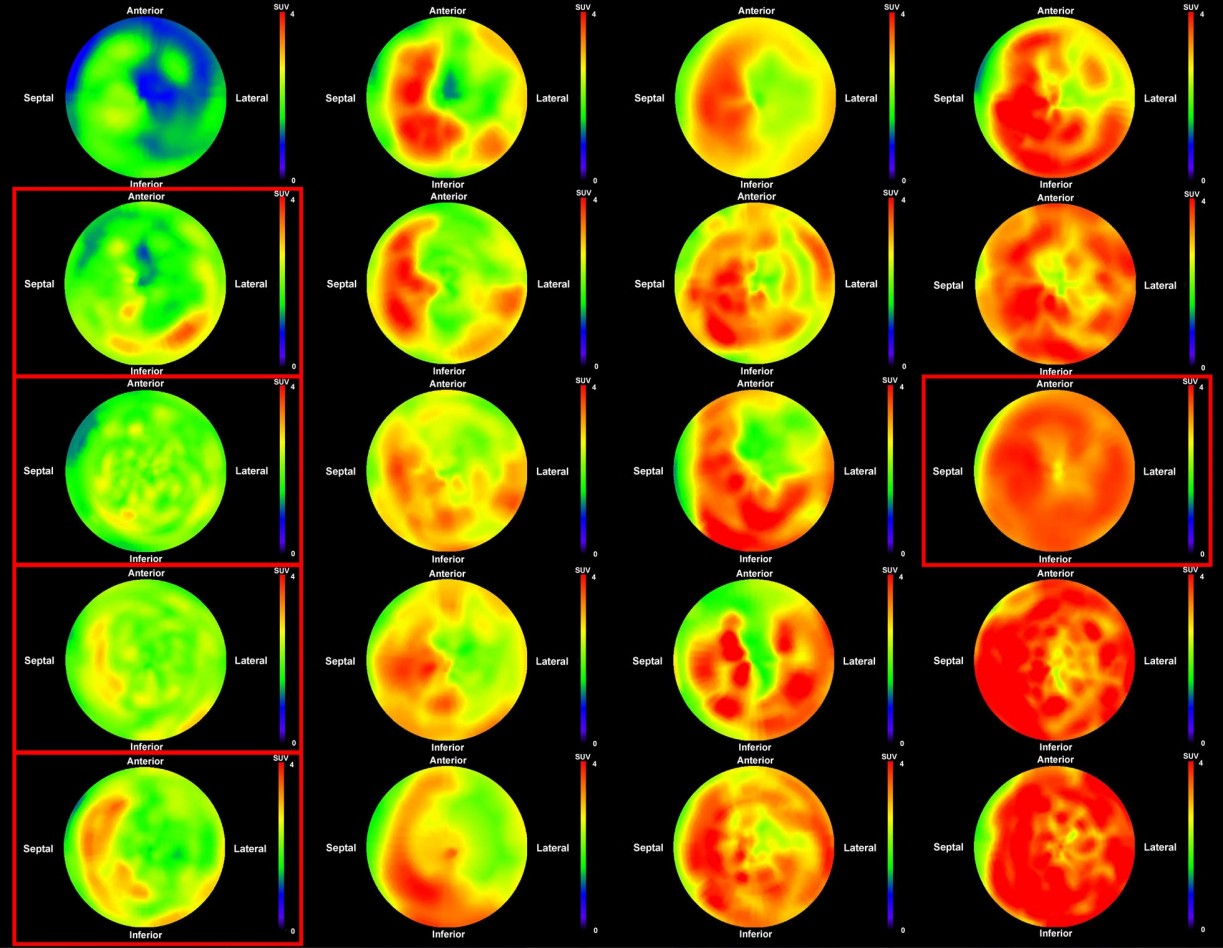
Polar maps of myocardial ^99m^Tc-PYP uptake in patients with transthyretin amyloid cardiomyopathy. Polar maps displaying regional SUV distribution in all 20 patients with transthyretin amyloid cardiomyopathy, sorted by ascending total cardiac SUV (top-left = lowest, bottom-right = highest) to visualize patterns associated with increasing amyloid burden. Red frames indicate cardiac transthyretin amyloidosis variant cases; unframed maps represent cardiac transthyretin amyloidosis wild-type. The upper SUV display limit is set to 4.0 for all maps. The center of the polar map represents the apex
